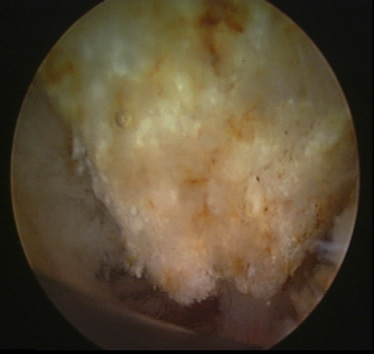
The review process took place after the training sessions and there was no further collaboration after the analysis had begun. In order to validate the classification system, we analyzed the intra- and inter-observer reliability of the system. Two independent observers, who were fellowship-trained sports surgeons, blinded to the clinical and magnetic resonance imaging (MRI) data were asked to classify the selected arthroscopic pictures in a random order and at three-week intervals in order to establish the intra-observer reliability. The inter-observer reliability was also analyzed from the results gathered in the first time of each observer. The condition of supraspinatus tendon was also recorded from the operative note as no tear, partial thickness tear, and full-thickness tear.
